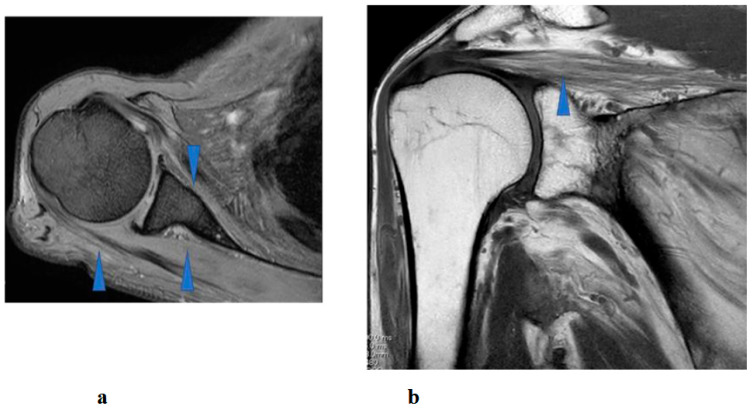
Right shoulder T1 and T2-weighted magnetic resonance imaging showed atrophy and fatty infiltration of several musculus, including supraspinatus, deltoideus, and subscapularis (arrow) on coronal (a) and sagittal (b).
